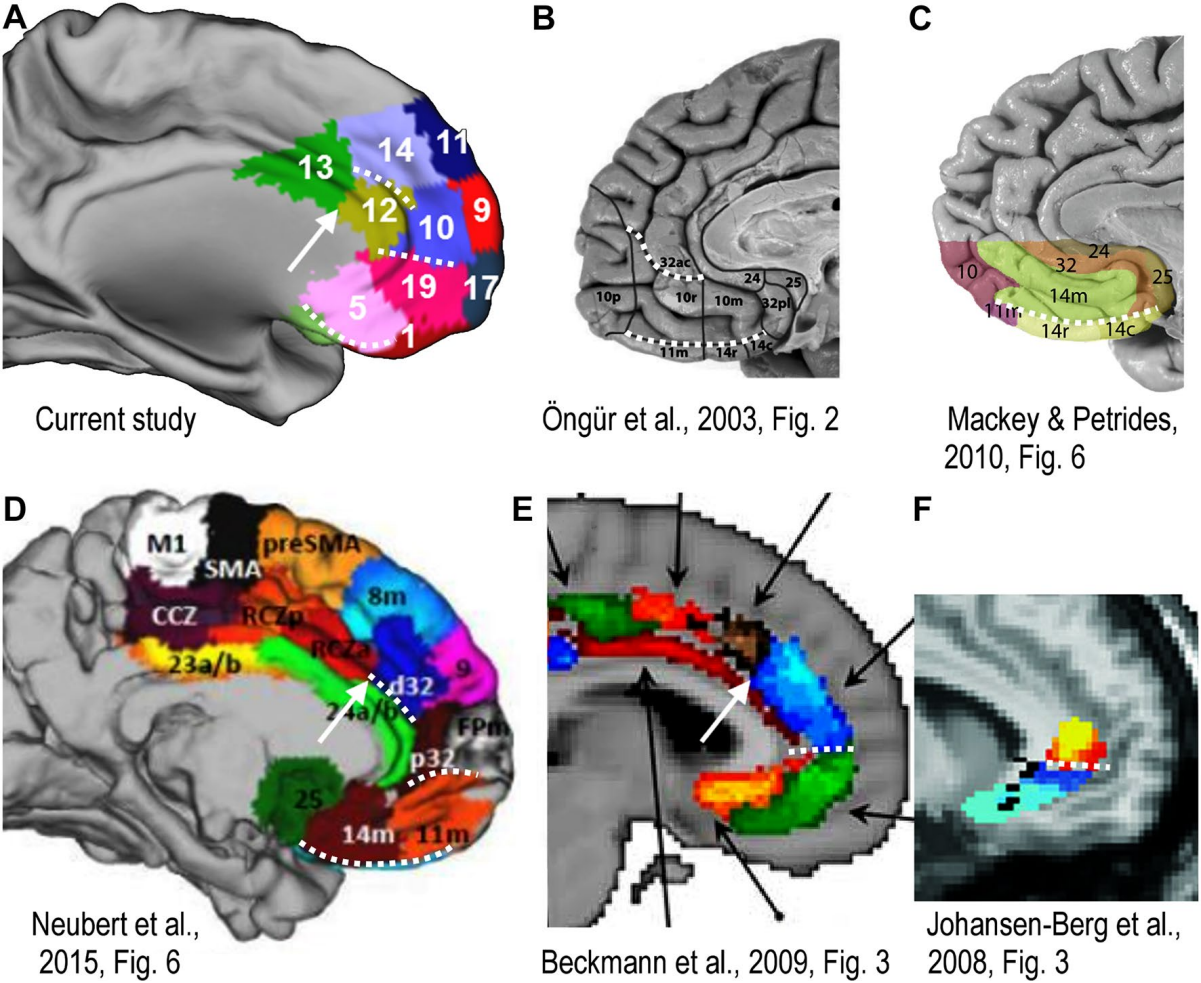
Comparison of parcellation schemes for the medial frontal surface from different studies and different modalities. *Dotted white lines* indicate boundaries between delineated areas that possibly correspond to the significantly co-localized replicable boundaries found in this study (e.g., Fig. 2a). *White arrows* in panels a, d and e mark potential border between MCC and ACC. *Black arrows* in panel e are in the original image and are not interpreted here. All pictures reproduced with permission. a Resting-state functional connectivity: data from the current study. b Cytoarchitectonic parcellation: Öngür et al. 2003. c Cytoarchitectonic parcellation: Mackey and Petrides, 2010. d DWI probabilistic tractography: Neubert et al. 2015. e DWI probabilistic tractography: Beckmann et al. 2009. f DWI probabilistic tractography: Johansen-Berg et al. 2008
